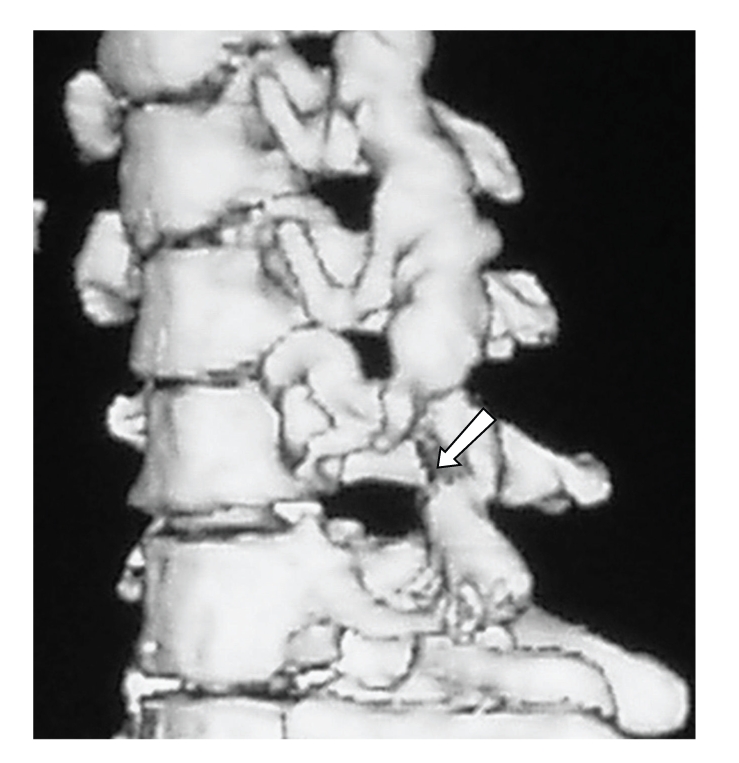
CT 3D reconstruction of the cervical spine shows erosion of the left intravertebral C6-C7 foramen (arrow).
